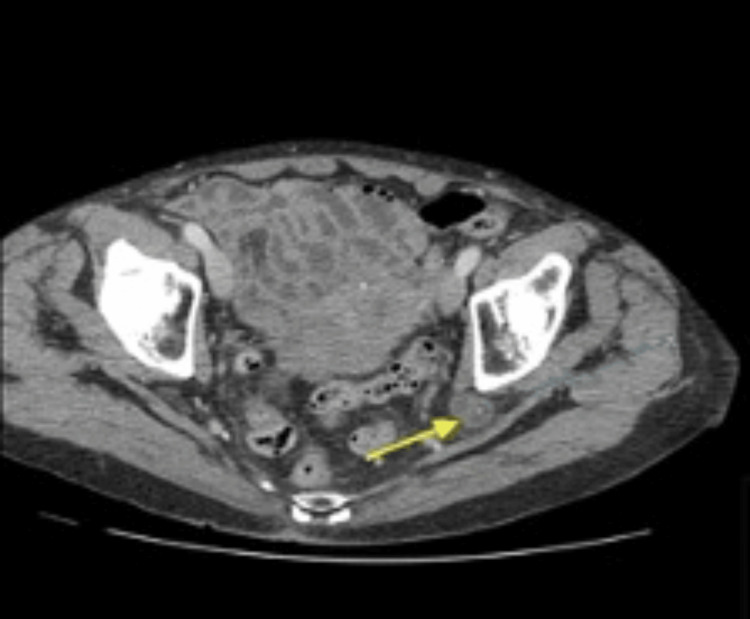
CT scan axial section demonstrates a dilated ureter outside the ureter. This figure is obtained from the author’s previous publication [4].
